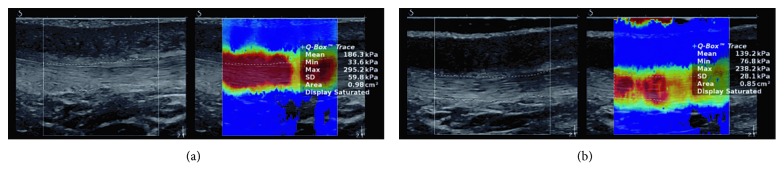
Quantitative evaluation of shear stress in the patella tendon using area evaluation of ultrasound images before (a) and after (b) the high-induction magnetic stimulation.
